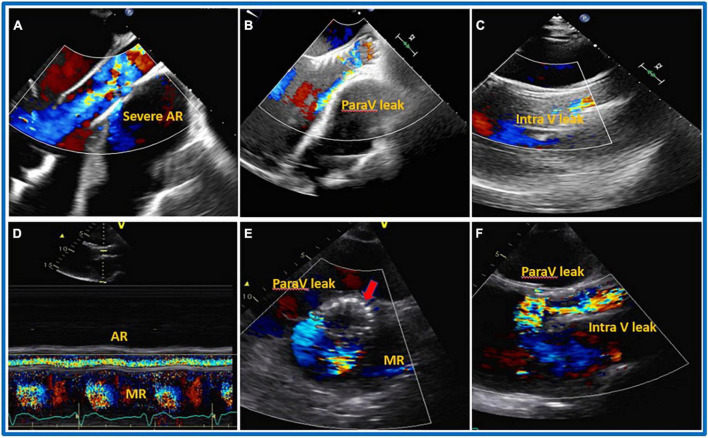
Transcatheter aortic valve implantation (TAVI) procedure. 32 years old gentleman with severe dilated cardiomyopathy, severe reduced systolic function (Ejection Fraction less than 10%), severe dilated and reduce systolic function of the right ventricle (RV), on left ventricular assist device (LVAD) as Bridge To Therapy (BTT) developed severe aortic regurgitation (AR). On transthoracic (TTE) the patients also had severely dilated RV, severe tricuspid regurgitation (TR) and associated severe liver and kidney dysfunction on hemodialysis. The patient underwent TAVI procedure with mild to moderate residual intravalvular AR. Five days after the procedure, TTE was repeated showing the presence of severe PV leak and the possible protrusion of the prosthesis into left ventricular outflow tract (LVOT). The patient died after 10 days from the procedure due to end stage heart failure and multi organ failure. (A) Pre-TAVI implantation, (B) TTE, mild to moderate paravalvular leak during TAVI procedure and before aortic valve (AV) deployment, (C) parasternal long axis TTE just after deployment, mild to moderate intra and paravalvular leak, (D–F) 5 days later S/P TAVR with likely severe intra and paravalvular leak, possible protrusion of the AV prosthesis into LV. (D) Color M-mode with AR in systole and diastole. (E) Shot axis. (F) Parasternal long axis.
